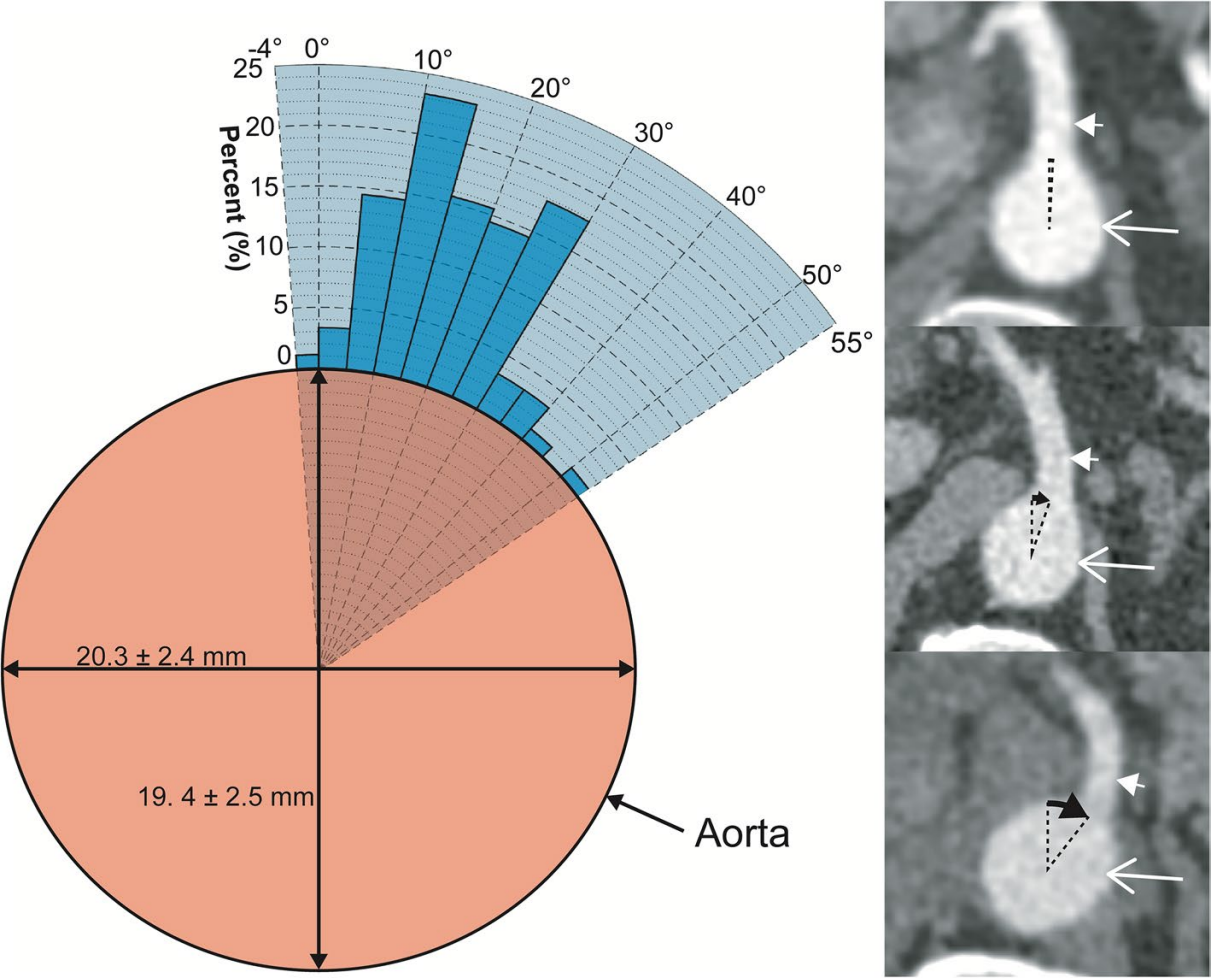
Clockwise angle of the origin of the coeliac trunk from the aorta in the axial plane. Histogram of the angles of origin of coeliac trunk from the aorta in the axial plane plotted as a percentage of participants. The minimum and maximum angle is represented by the radial range of the graph (− 4 to + 55°; light blue shading). The cross-sectional dimensions of the aorta at the level of the coeliac trunk are also included. Inset CT images show examples and calculations for the coeliac trunk clockwise angle from participants in the (top to bottom) lowest, median and highest decile. Long white arrow, aorta; short white arrow, coeliac trunk; black arrow, measured angle
